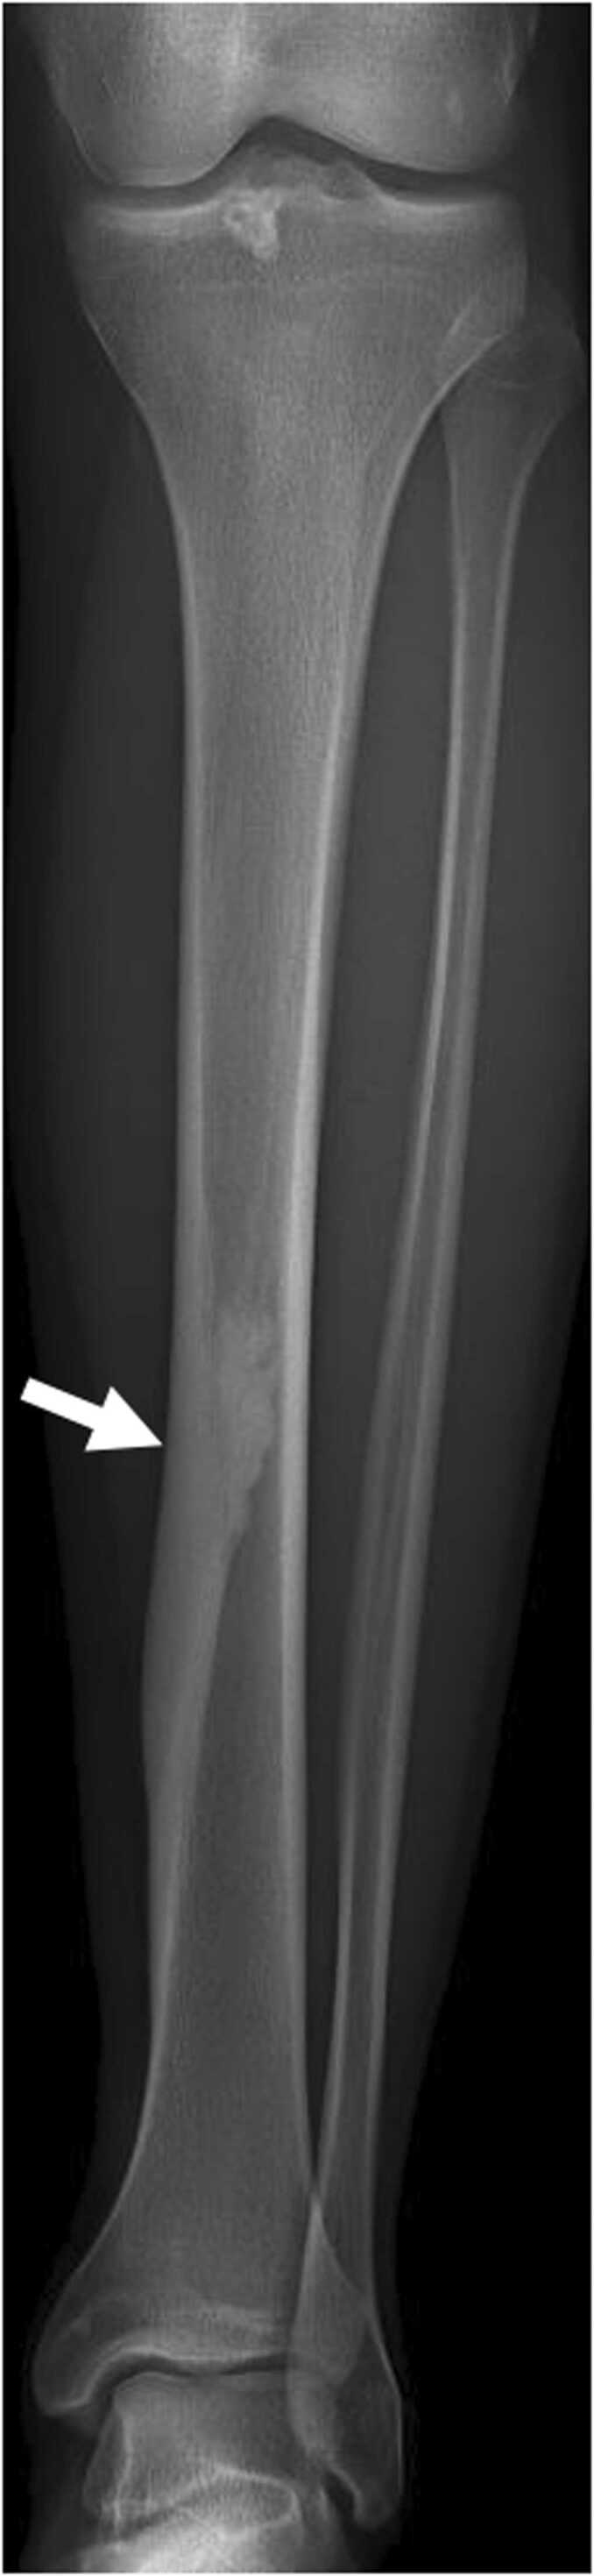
Melorheostosis in a 20-year-old young man with swelling of medial side of the left leg. Face radiography of the left leg showing endostotic hyperostosis of the middle-third of tibial diaphysis giving “melting wax flowing down the side of a candle” appearance (white arrow).
